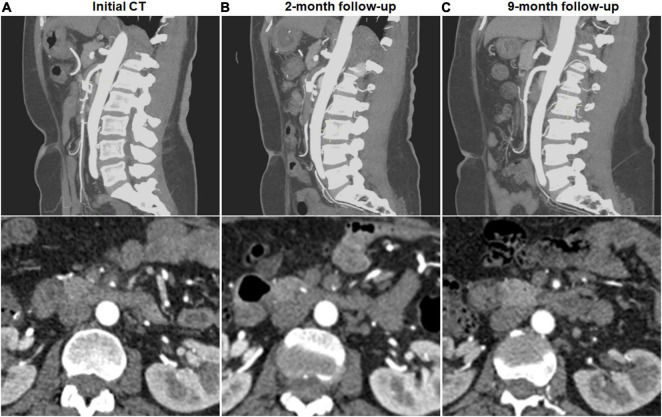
Complete remodeling of type III spontaneous isolated superior mesenteric artery dissection on follow-up computed tomography (CT) angiography. (A) Initial CT angiography showed the thrombosed false lumen with ulcer-like projection (ULP) in a 52-year-old woman. (B) Two months later, stenosis of true lumen was aggravated with thrombosis of false lumen and ULP. (C) Nine months later, ULP disappeared and thrombosis in the false lumen was completely resolved.
